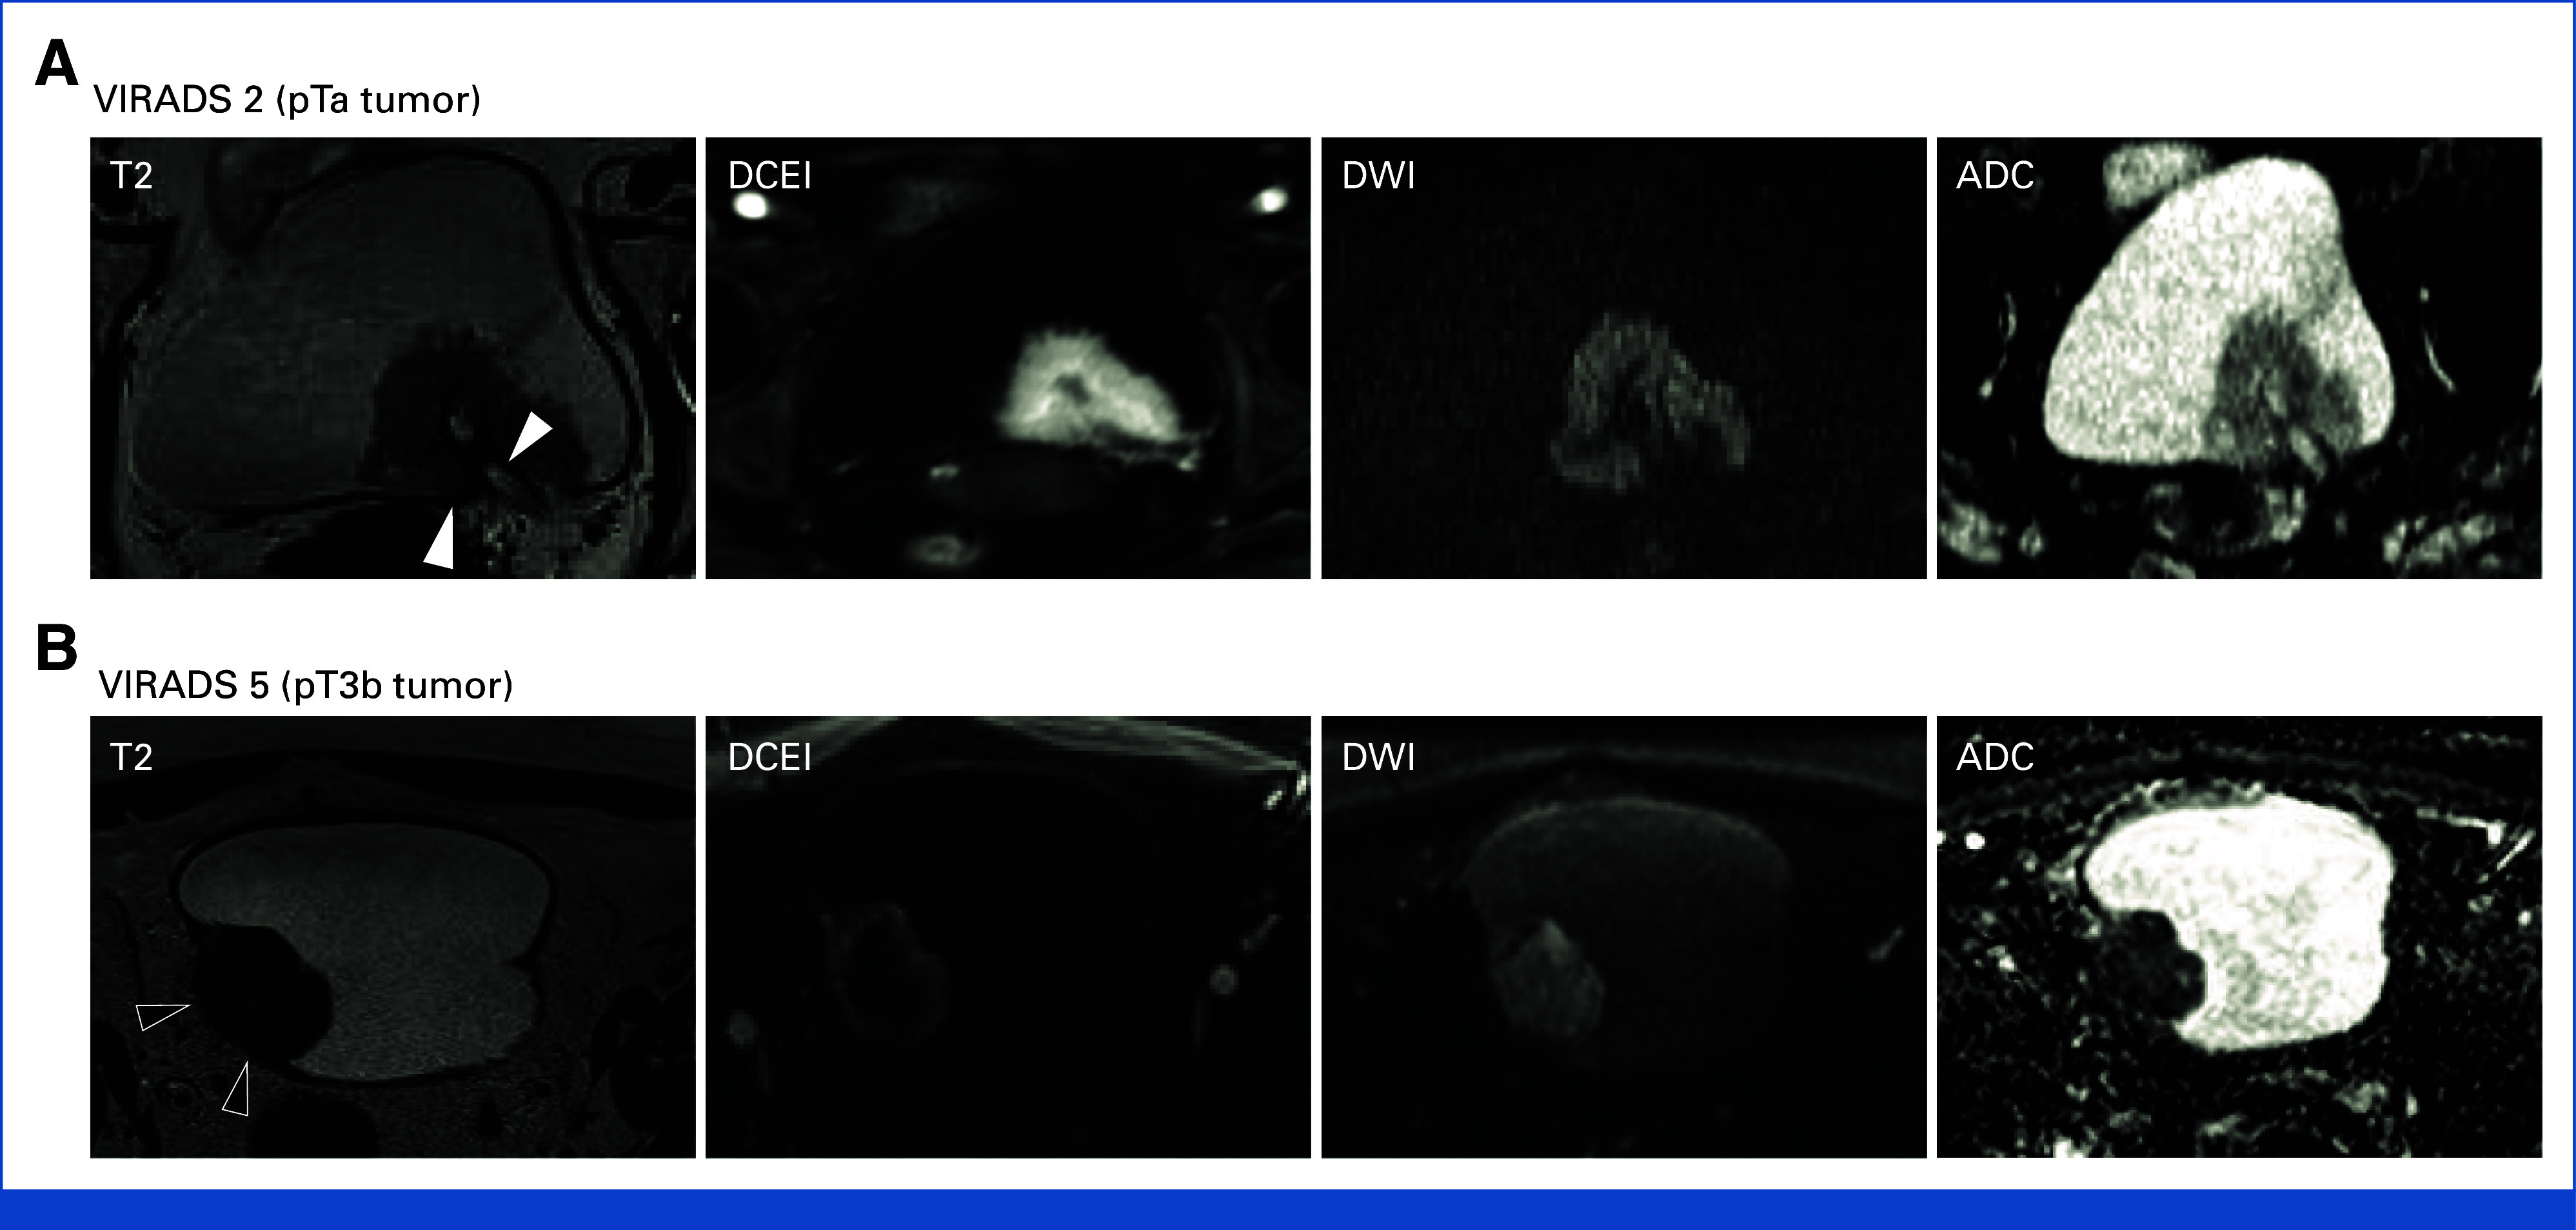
Examples of mpMRI images from recruited participants for (A) noninvasive (pTa) and (B) invasive (pT3b) bladder cancers initially thought to be possible MIBC (Likert 3-5) at flexible cystoscopy. White arrows indicate intact muscularis propria. Black arrows indicate loss of normal muscularis propria because of invasive tumor. For the full NIHR Health Technology Assessment report, please refer to the study by James et al.^37^ ADC, apparent diffusion coefficient; DCEI, dynamic contrast enhancement image; DWI, diffusion-weighted imaging; MIBC, muscle-invasive bladder cancer; mpMRI, multiparametric magnetic resonance imaging; NIHR, National Institute for Health Research; T2, MRI T2 weighted; VIRADS, Vesical Imaging-Reporting and Data System.
